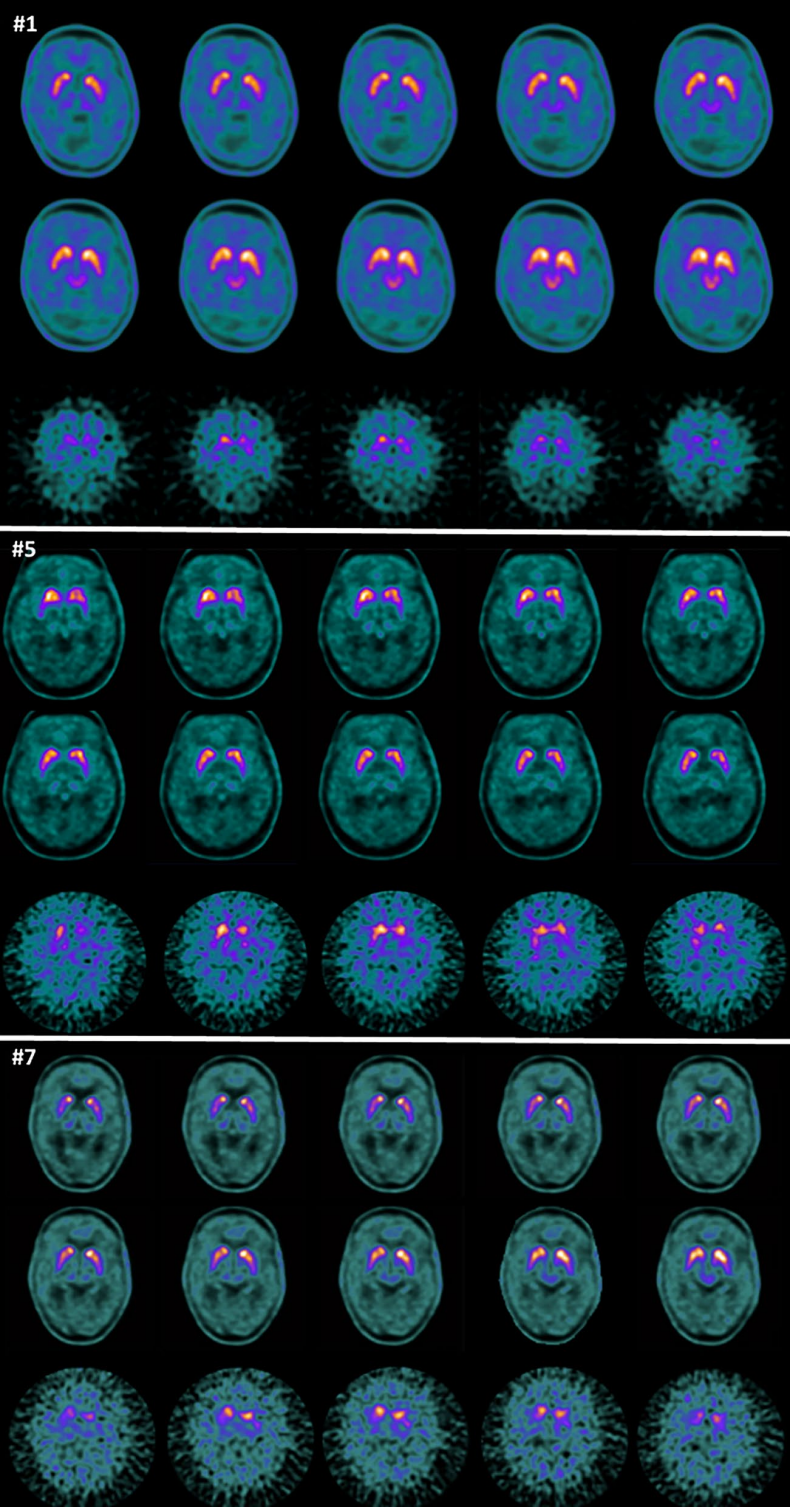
Representative transverse images of the striatum on FDOPA-PET (2 top rows for each case) and DAT-SPECT (bottom row for each case) for patients #1, #5 and #7. For all three cases, FDOPA-PET was reported normal and DAT SPECT abnormal
